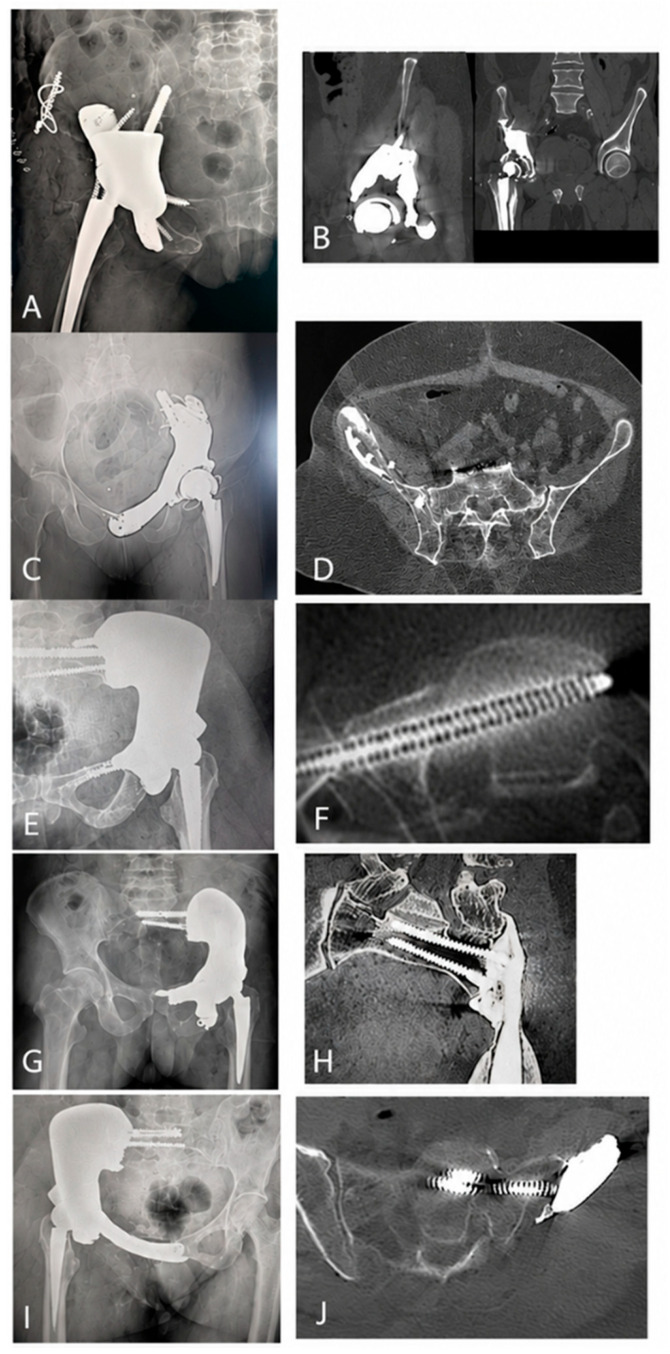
Postoperative radiographs and CT scans obtained at 6 weeks and 6 months, respectively, for each type of pelvic reconstruction according to WChC: WChC-Aa (A,B), WChC-Ab (C,D), WChC-Ba (E,F), WChC-Bb (G,H), and WChC-C (I,J).
